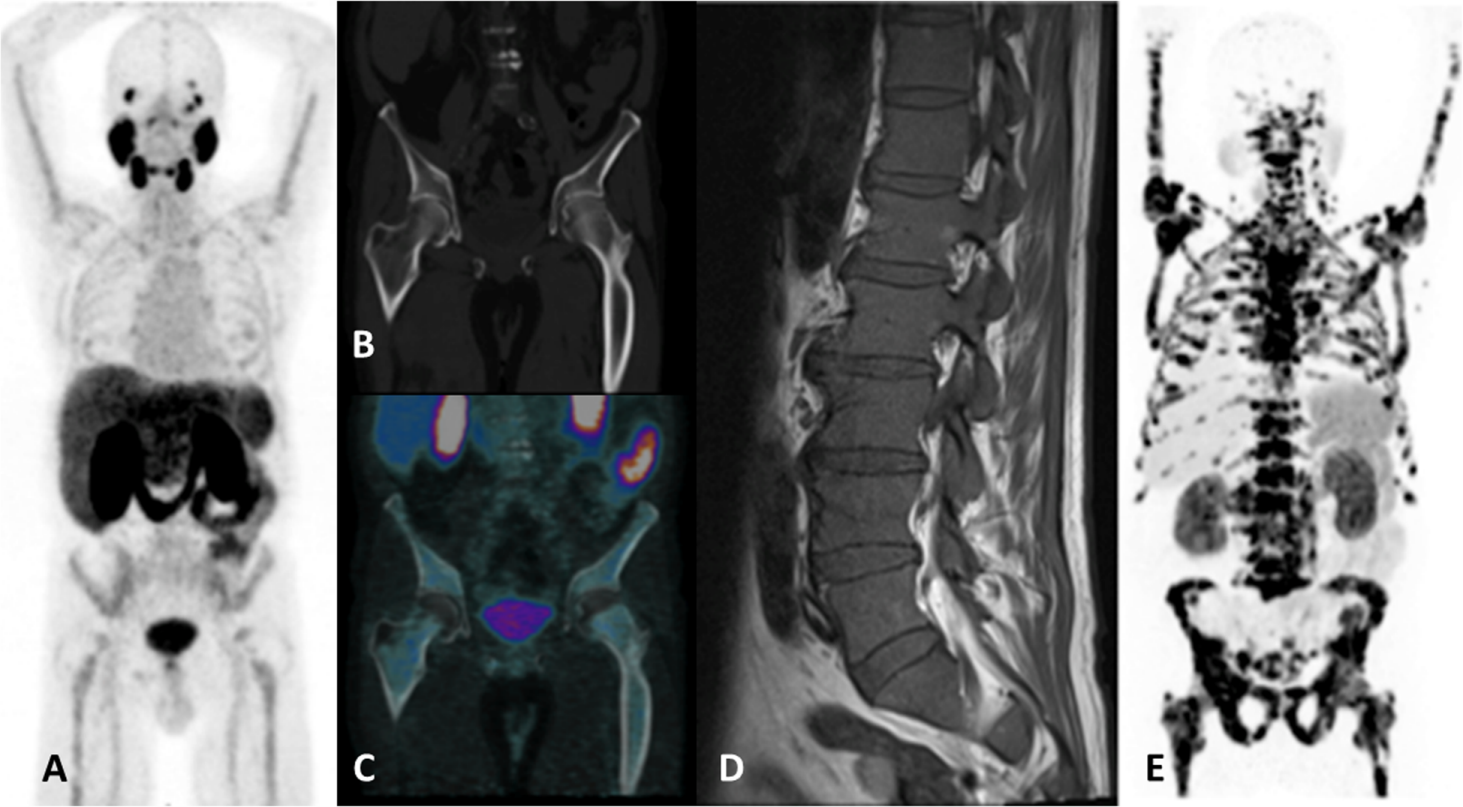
Patterns of diffuse bone PSMA uptake in two different patients. ^68^Ga-PSMA-PET/CT MIP (a), coronal CT (b) and coronal PET/CT (d) images show dense and inhomogeneous bone marrow throughout the skeleton with a discrete diffuse uptake, in a patient with a previous diagnosis of myelodysplasia syndrome. A T1w (d) MRI corroborates showing a diffuse infiltration of the bone marrow. A neoplastic infiltration in a different patient is shown on another ^68^Ga-PSMA-PET/CT MIP (e) to highlight the uptake pattern differences
